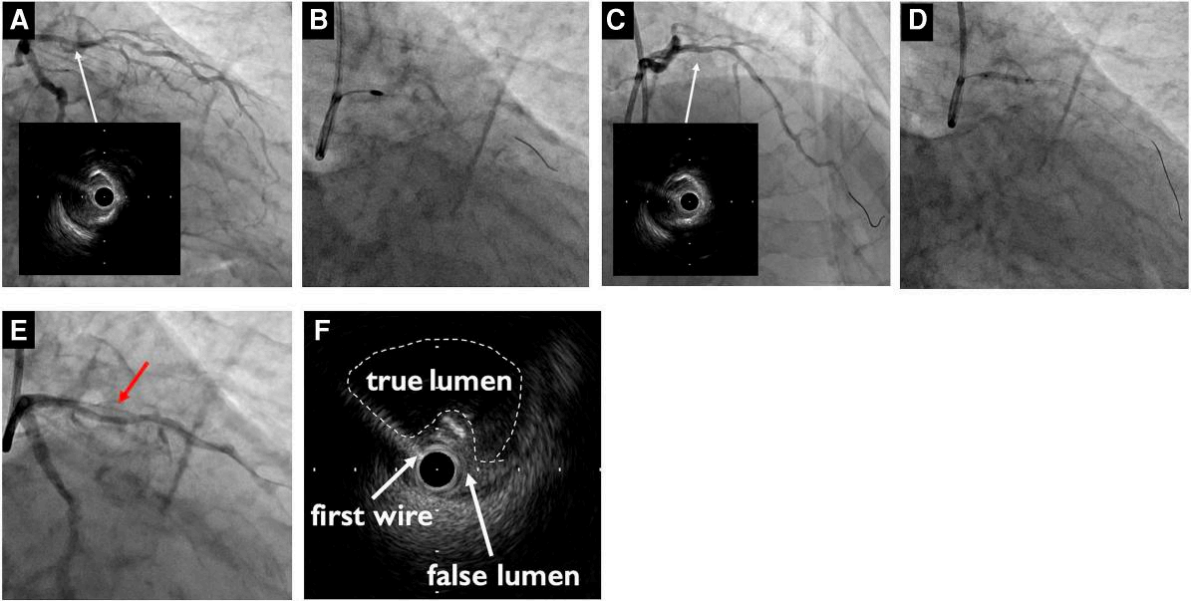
Percutaneous coronary intervention. (*A*) Angiography and intravascular ultrasound showing circumferential superficial calcification (>180° arc) (white arrow). (*B*) Rotational atherectomy performed with a 1.5 mm Rota Pro burr at 180 000 rpm. (*C*) Luminal gain achieved after atherectomy with no dissection (white arrow). (*D*) High-pressure scoring (cutting) balloon angioplasty. (*E*) The first guidewire migrated into a false lumen behind the dissected calcified segment (red arrow). (*F*) Intravascular ultrasound image obtained over the first guidewire located in the false lumen, demonstrating the spatial relationship between the true and false lumens.
